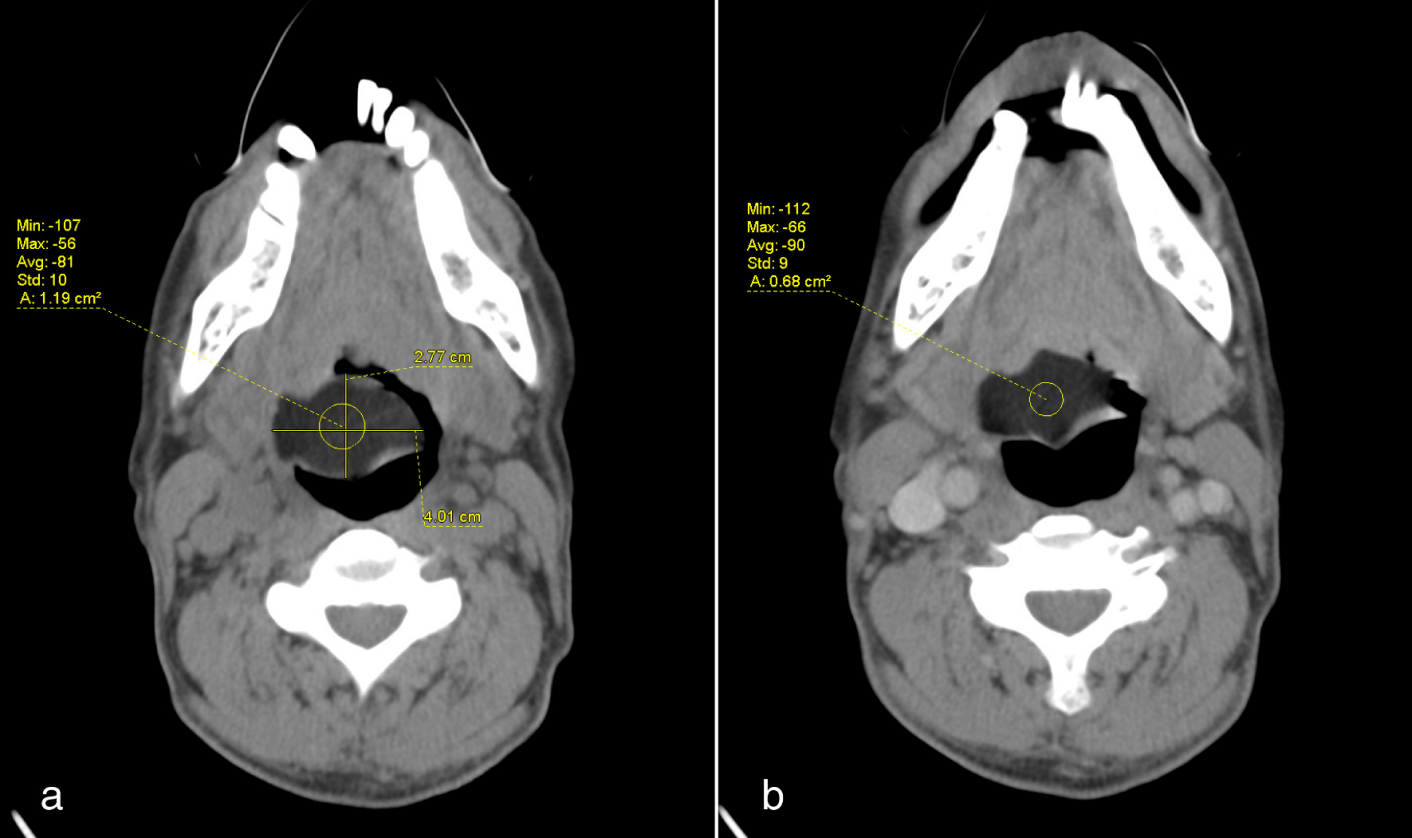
(a) Plain and (b) contrast-enhanced CT scan showing a hypodense lesion at the right vallecula with mean Hounsfield unit of −81, suggestive of fat content. No significant enhancement is seen post contrast.
